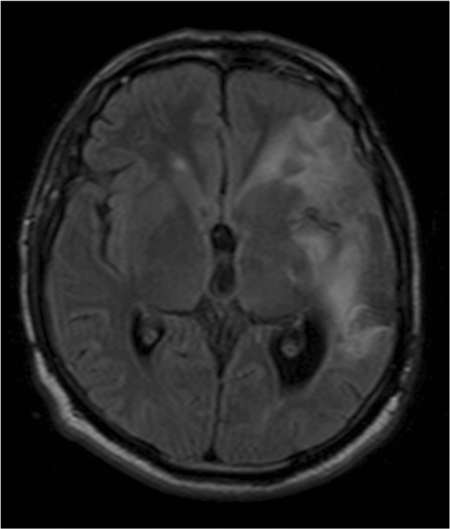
The extent of the infarction was demonstrated by FLAIR MRI sequence
